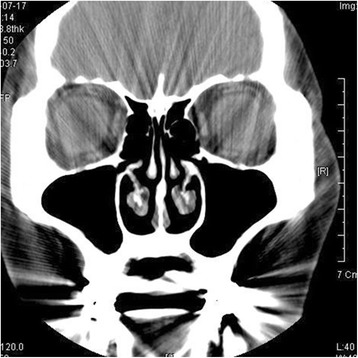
Computed tomography (CT) view at 3.5 months follow-up. Radiolucency is shown on both sinuses and all clinical symptoms of right side sinusitis have been relieved
